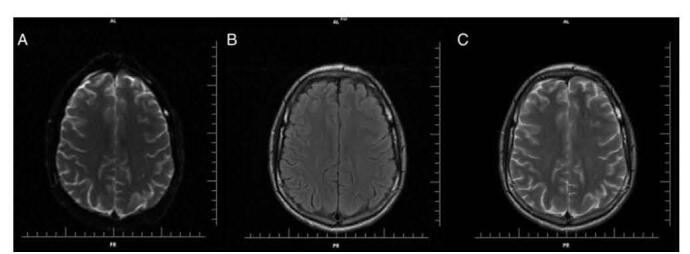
Brain MRI osteosarcoma (30 days after onset). (A) DWI. (B) Axial T2/Flair. (C) Axial T2. Adapted from Valle et al. (2016). Stroke-like encephalopathy following high-dose IV MTX in an adolescent with osteosarcoma: a case report^[7]^.
